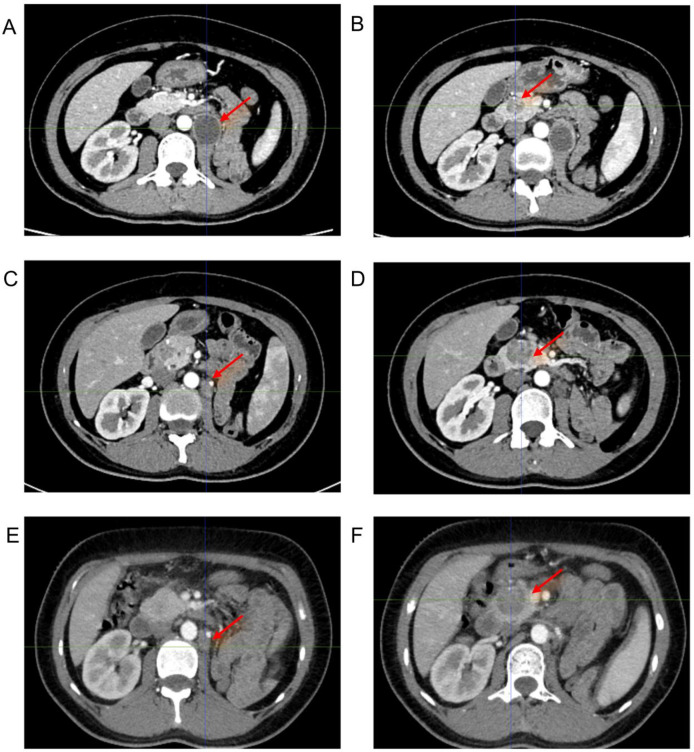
Contrast-enhanced CT before oral olaparib administration and two follow-up contrast-enhanced CT scans after drug therapy. (A, B) In August 2025, prior to treatment with olaparib, the tumor in the renal region was large, measuring 3.6 x 2.8 cm, and the tumor in the pancreatic head measured 2.3 cm; (C, D) November 2025: The renal tumor measured 2.0 x 1.2 cm, and the pancreatic head tumor measured 2.6 x 2.4 cm; (E, F) December 2025: The renal tumor had decreased slightly in size compared to November, and the pancreatic head tumor measured 2.7 x 2.6 cm.
